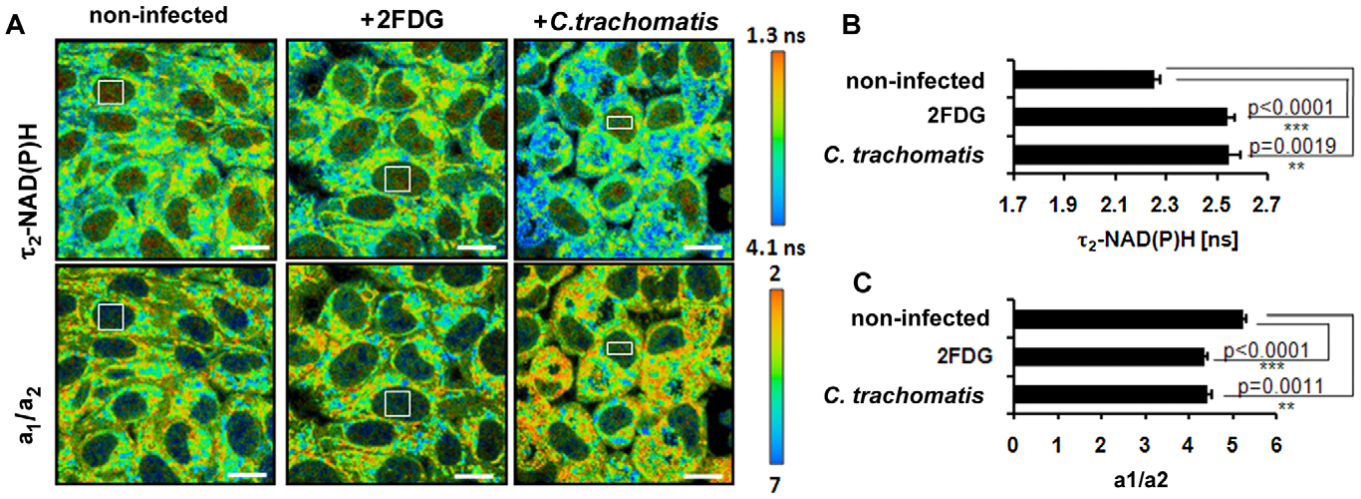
Cellular starvation in *C. trachomatis*-infected cells. HEp-2 cells were left untreated (control) or were treated with 5 mM 2FDG or were infected with *C. trachomatis* for 24 hours. (A) Upper panels: Color-coded images of τ_2_-NAD(P)H. Lower panels: Color-coded images of the a_1_/a_2_ ratio. White squares inside the nucleus show representative ROIs used for quantitative analysis (scale bar = 20 µm). (B) Treatment of cells with 2FDG or infection with *C. trachomatis* increases τ_2_-NAD(P)H inside the nucleus. Quantitative analysis of τ_2_-NAD(P)H (n = 54 ROIs from three independent experiments; mean±SEM). Detailed results of statistical analysis are shown in Table S5A. (C) Treatment of cells with 2FDG or infection with *C. trachomatis* decreases the a_1_/a_2_ ratio inside the nucleus. Quantitative analysis of a_1_/a_2_ ratio (n = 54 ROIs from three independent experiments; mean ±SEM). Detailed results of statistical analysis are shown in Table S5B.
